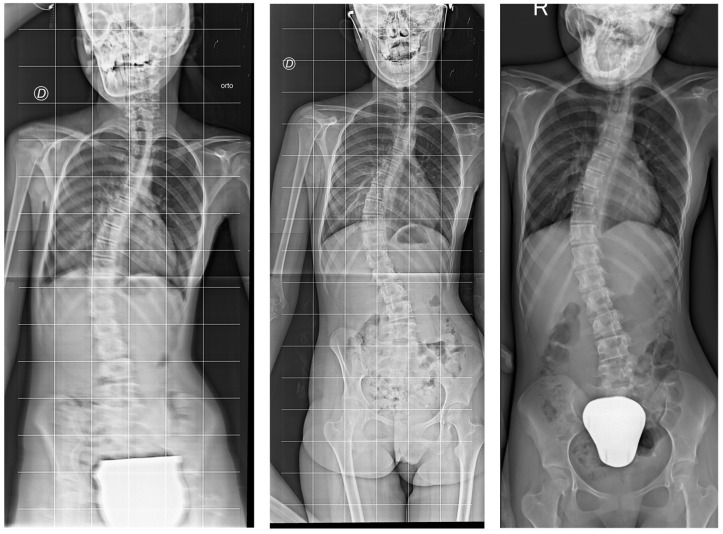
From (left) to (right), radiographs of participants no. 18 were collected at T0, T1, and T2, respectively. Letters “D” and “R” explicitate the right side of the body.
